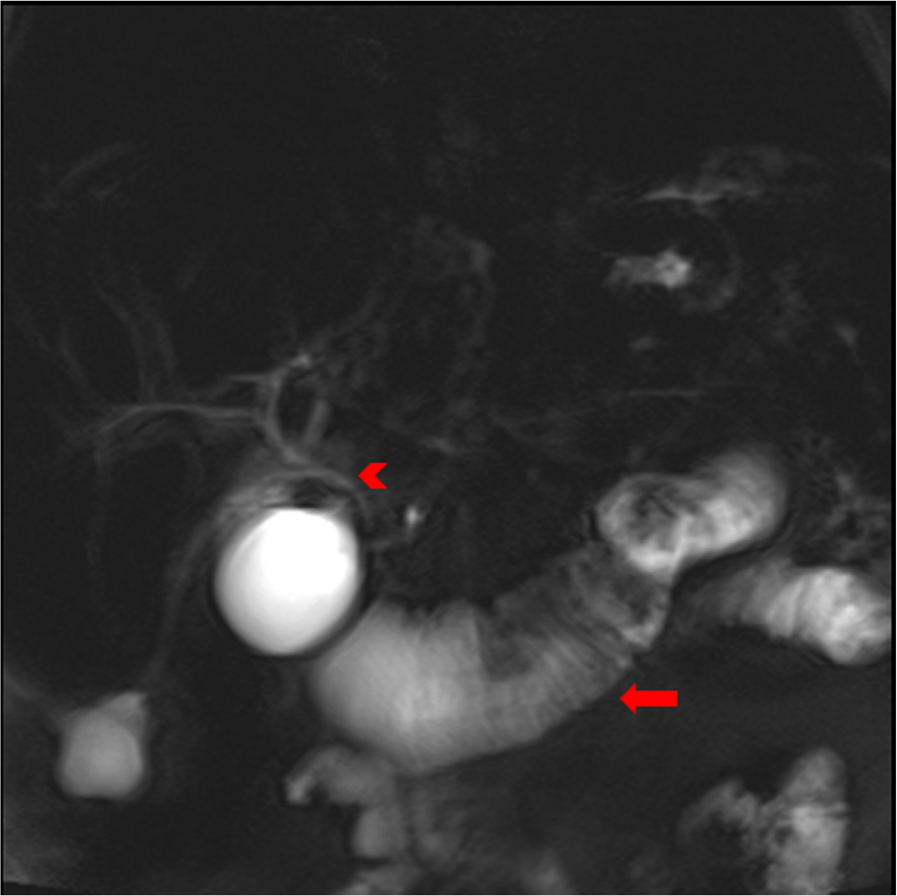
At magnetic resonance cholangiopancreatography, coronal T2-weighted half-Fourier acquisition single-shot turbo spin-echo image shows a markedly dilated afferent duodenal limb (*arrow*) and a non-dilated, normal-appearing biliary tree (*arrowhead*)
